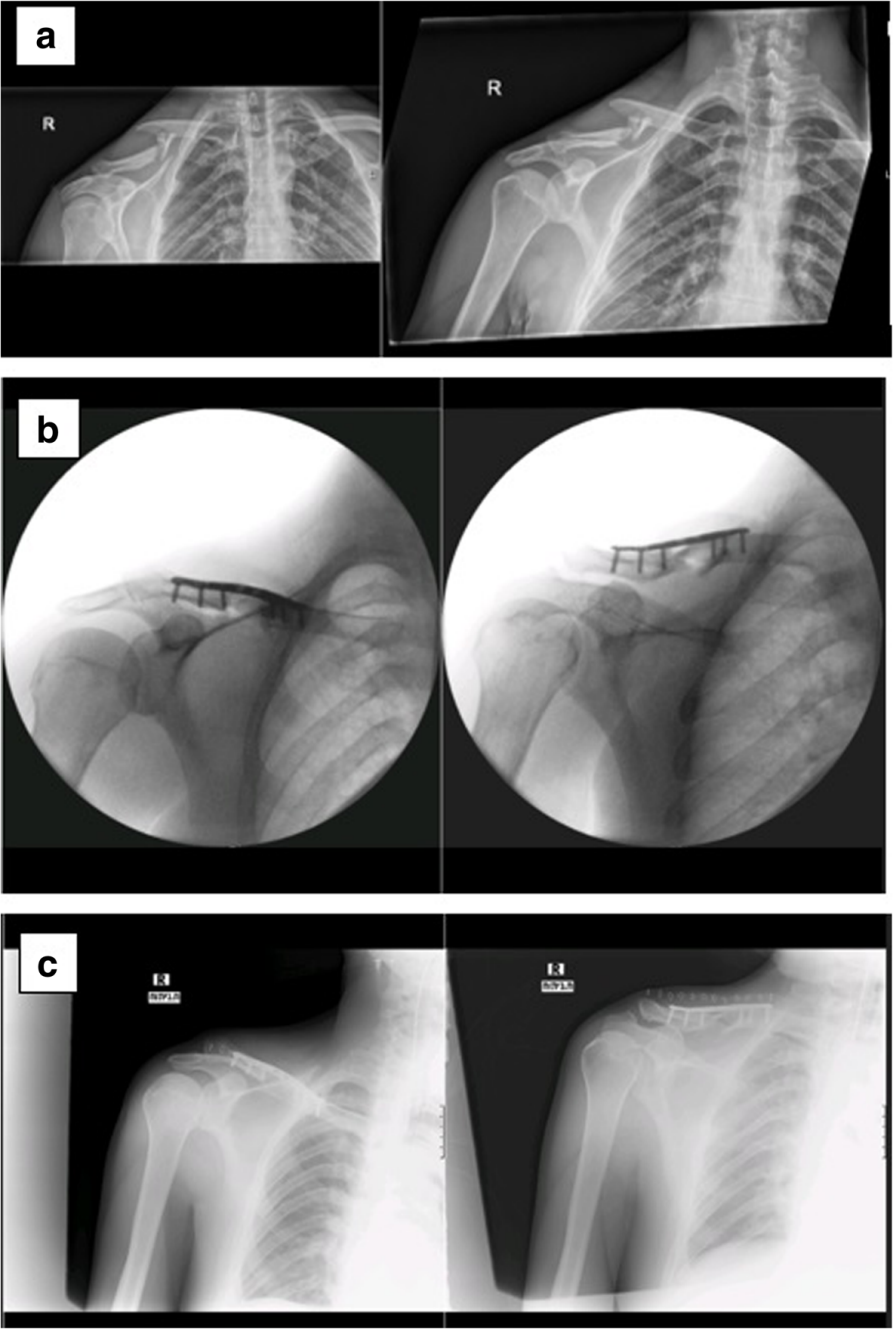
a X-ray: displaced comminuted right midshaft clavicle fracture; b intraoperative imaging; c postoperative X-ray with reduction and bridging osteosynthesis with an anatomical contoured locked plate
